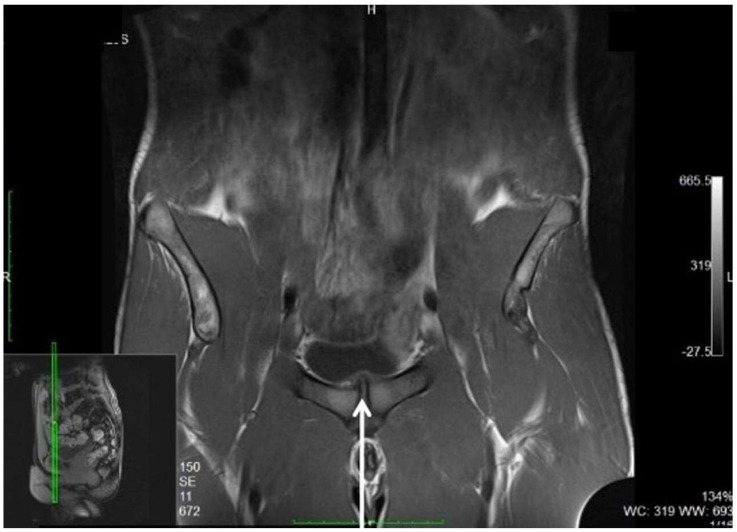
Coronal T1-MRI showing a symphyseal central disc protrusion (arrow).
